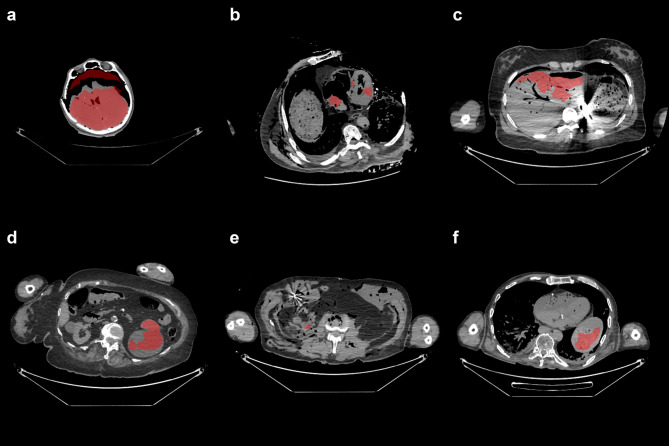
Representative examples of failed or inaccurate AI-based organ segmentations in postmortem CT data. For visualization purposes, single representative CT slices from 3D segmentations are shown for (a) brain, (b) heart, (c) liver, (d) left kidney, (e) right kidney, and (f) spleen. Segmentation errors were observed in cases with postmortem decomposition (b, c), traumatic injury (e), thermal injury, and other postmortem or pathological changes affecting tissue morphology. Such conditions may impair organ delineation and lead to inaccurate CT-derived organ weight estimation.
